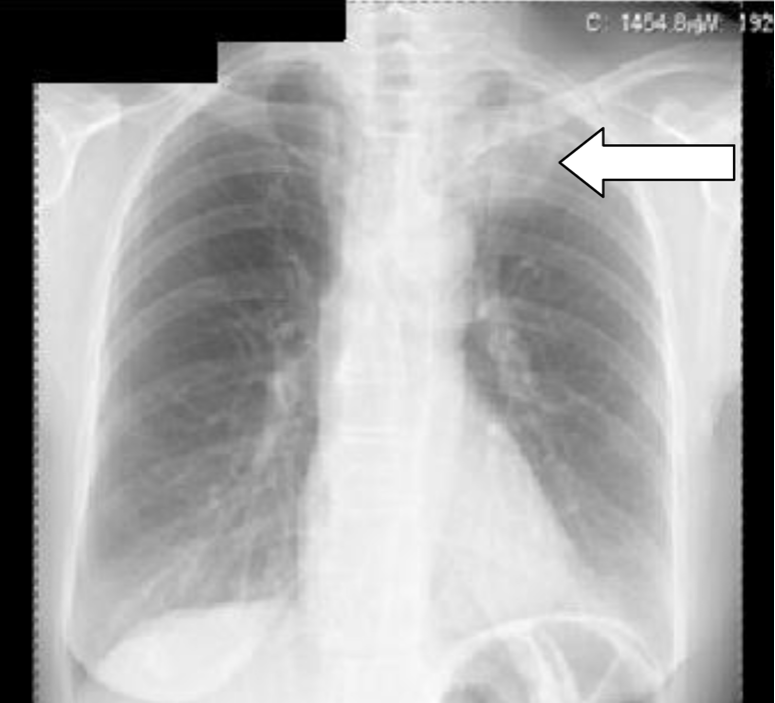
Example of grade 3 RP in the apical-lateral region of the left lung on chest x-ray. (= total score 3).
